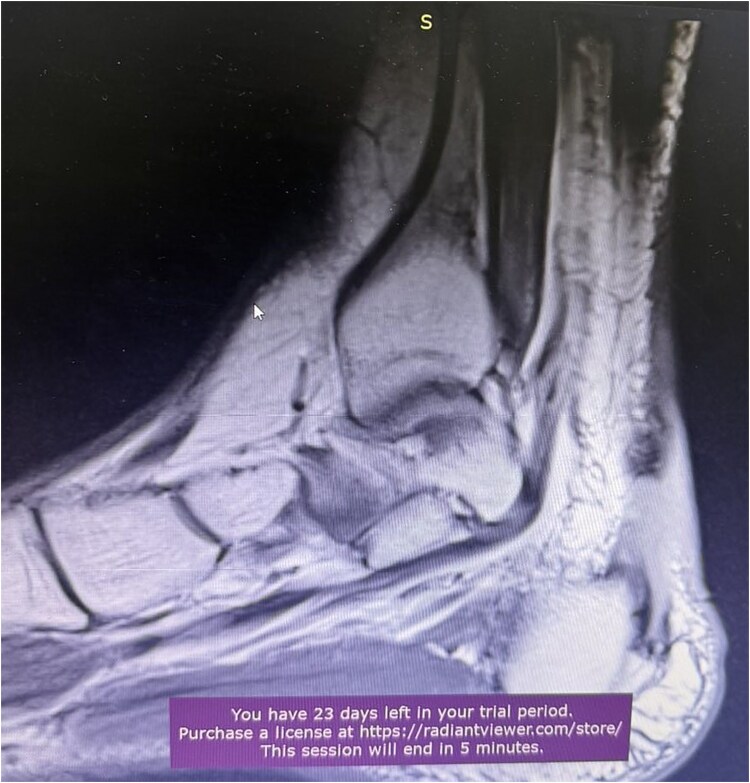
MRI of Achilles tendon rupture.
